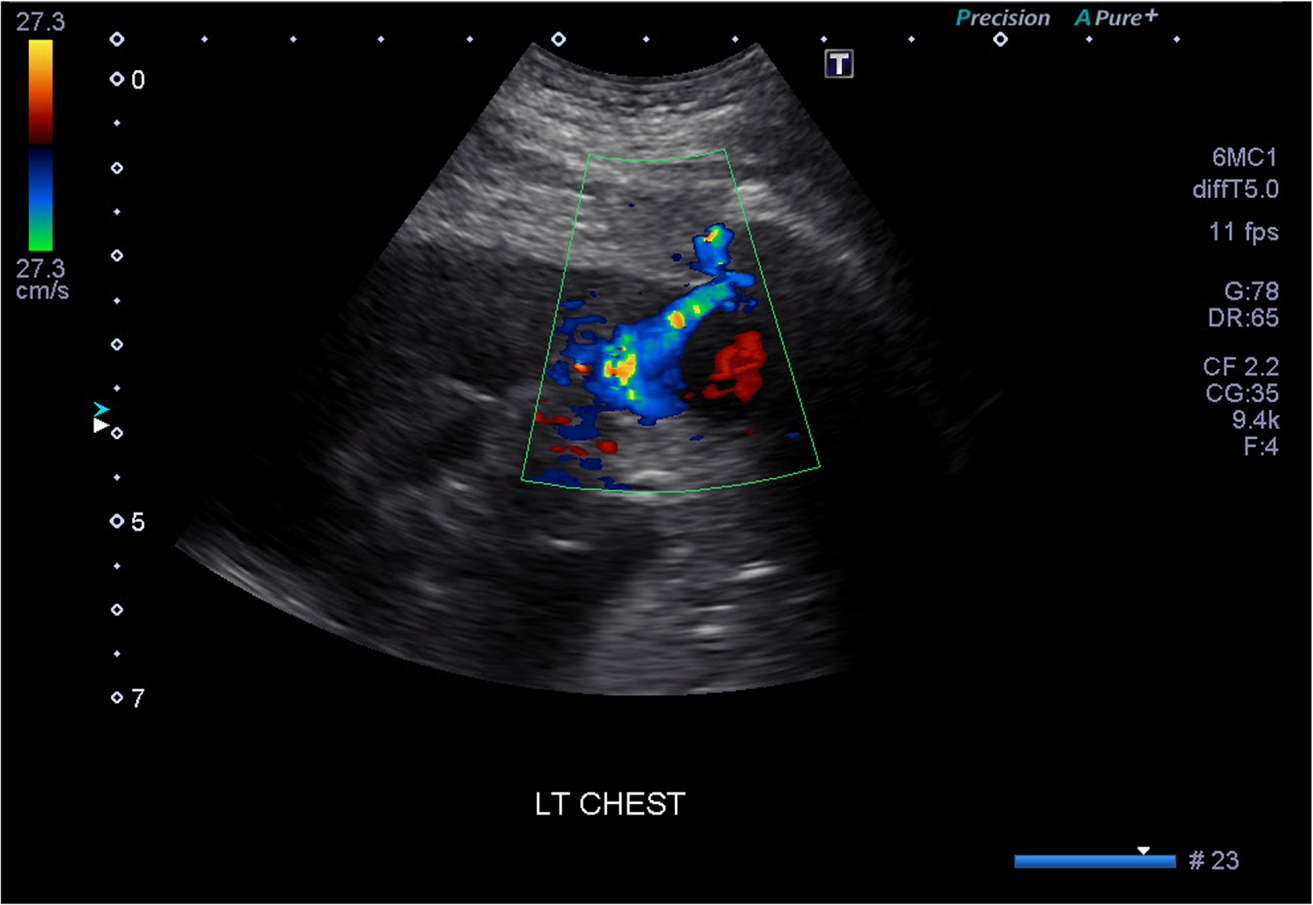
Color Doppler ultrasound image of the intercostal artery pseudoaneurysm. Note the characteristic yin-yang (red-blue) blood flow present in the pseudoaneurysm
